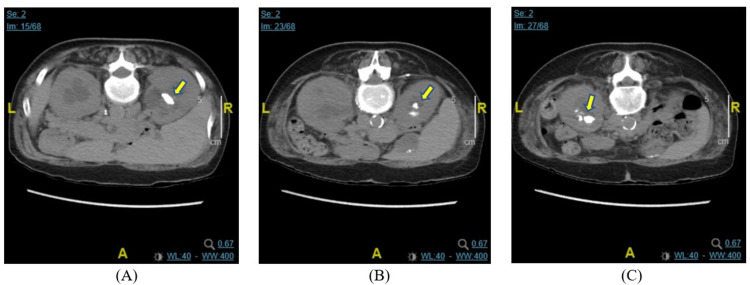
CT abdomen/pelvis without IV contrast in November 2020 Multiple stones within the right kidney with the largest ones measuring 1.8 cm in the upper pole (A) and an 8-mm stone within the lower pole (B). In the left kidney, there are multiple stones within the lower pole with the largest one measuring 1.5 cm (C) (arrows) CT: computed tomography; IV: intravenous
